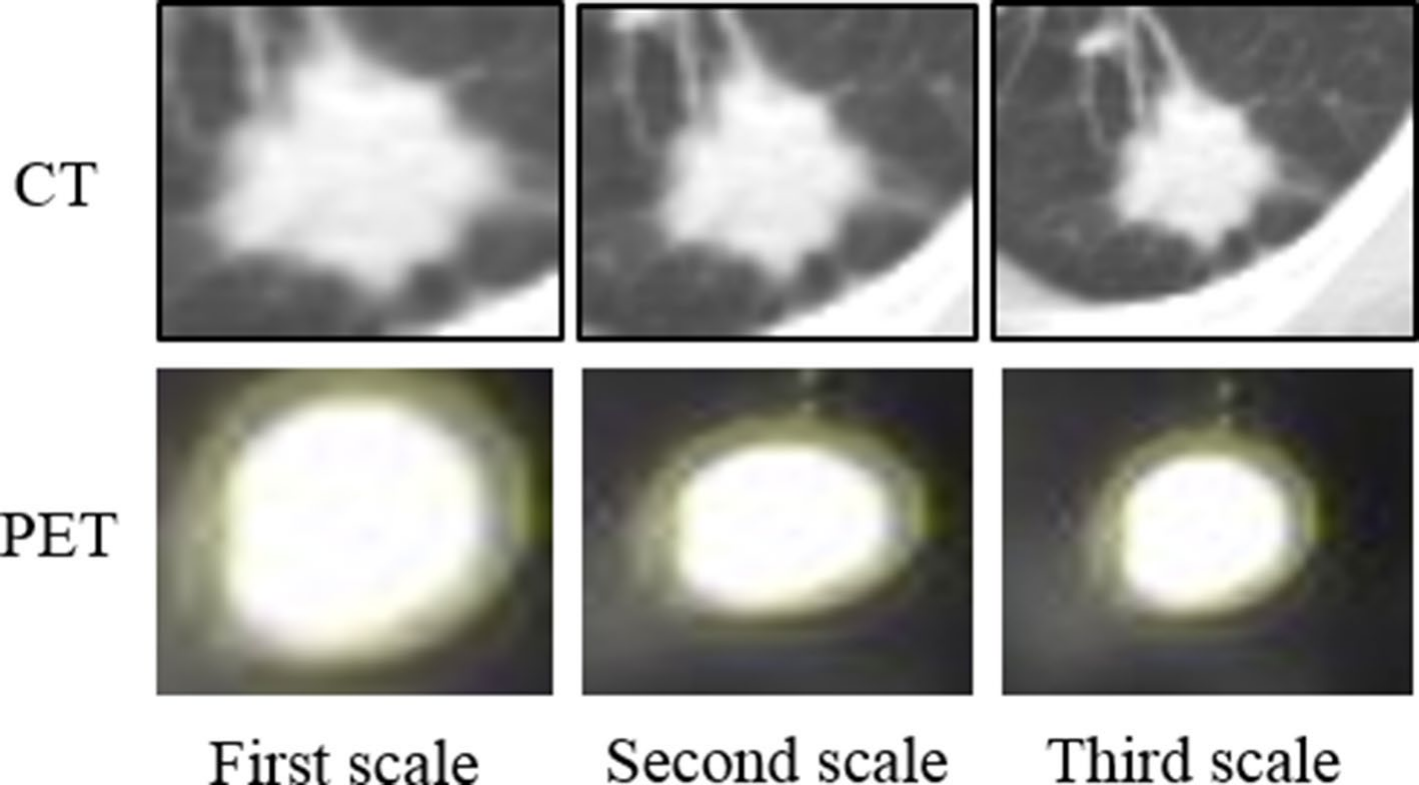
The CT and PET components cropped from three scales. These scales are stacked together and fed to the network.
